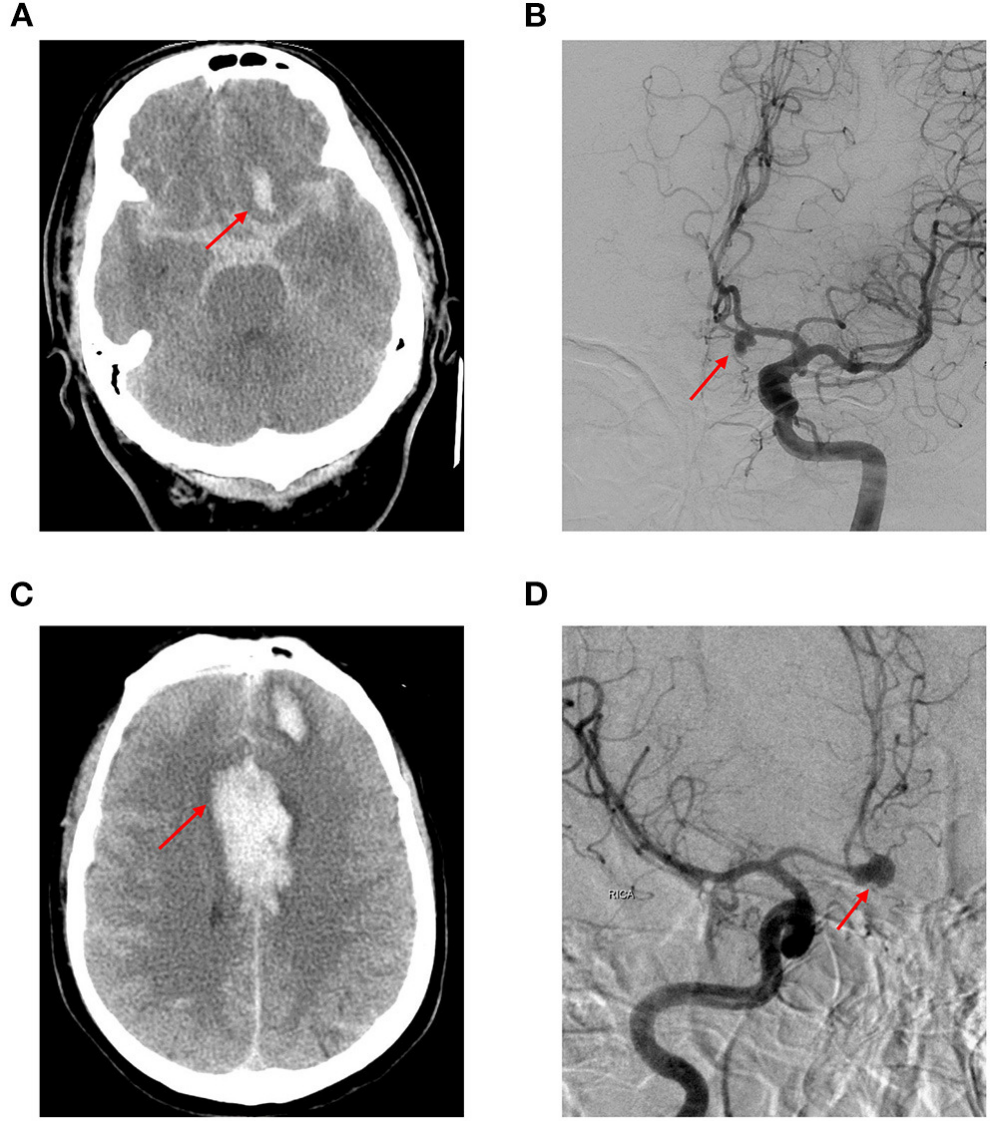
A 62-year-old Taiwanese woman presented to our emergency department with an abrupt and thunderclap headache. Initial cerebral computed tomography showed diffuse subarachnoid hemorrhage. (A) The subsequent digital subtraction angiography confirmed anterior communicating artery aneurysm (B); she was sent for clipping surgery and discharged 1 month after the condition was stabilized. Eight months later, she was readmitted due to altered consciousness during the routine morning jogging. Cerebral computed tomography showed intra-cerebral hemorrhage and subarachnoid hemorrhage (C); subsequent digital subtraction angiography demonstrated aneurysmal ruptured at the same location (D) (red arrow).
